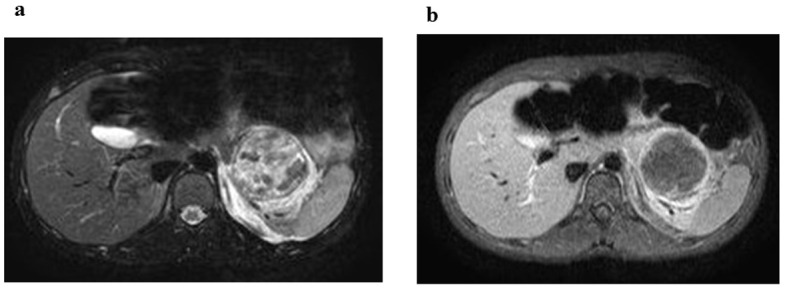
MRI reveals a mass approximate 50 mm in size in the pancreas. (a) T2-weighted MRI reveals a mass, measuring 50 mm approximately, in the pancreatic tail with associated heterogeneous high intensity that indicates the presence of an encapsulated solid and cystic mass with areas of hemorrhagic degeneration. (b) Dynamic contrast-enhanced MRI demonstrates contrast enhancement in the early arterial phase; the contrast enhancement gradually increases in the late phases.
